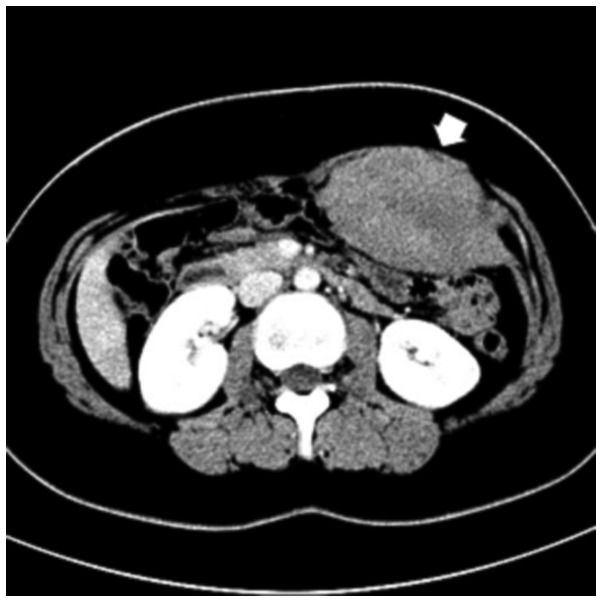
Computed tomography scan with contrast enhancement demonstrating the desmoid tumor originating from the abdominal transversal and internal oblique muscle fascia, with an inhomogeneous formation. Arrow indicates tumor.
